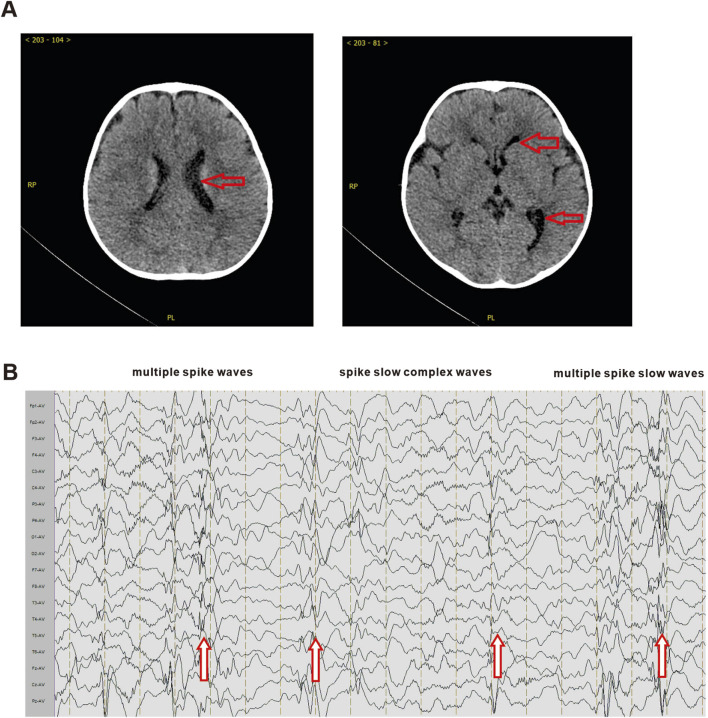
Brain CT and electroencephalogram of proband. (A) The brain CT scan results of the prband. Red arrows indicate the enlarged left lateral ventricle. (B) The EEG results of the proband. Red arrows indicate multiple spike waves, spike slow complex waves and multiple spike slow waves.
